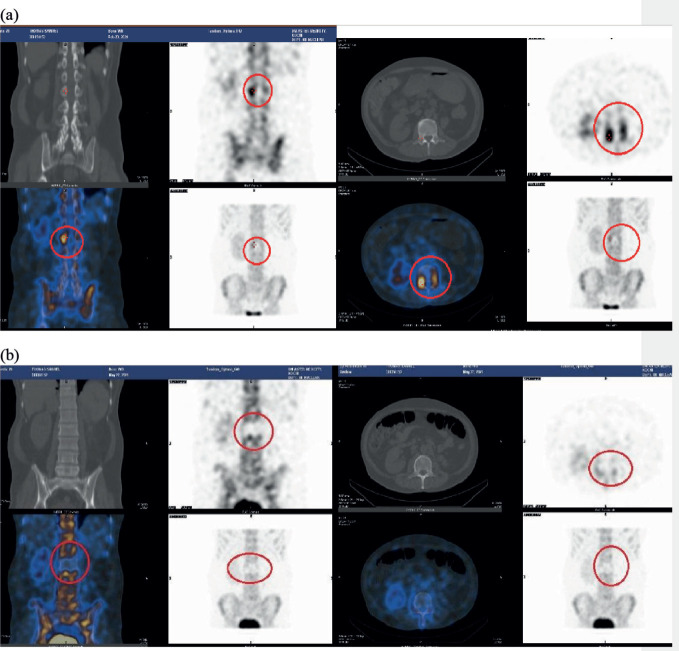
(a): SPECT images in metastatic RCC showing increased tracer uptake over the L2 pedicle on the right. (b): SPECT images 3 months after intracranial FSR, showing no tracer uptake over the L2 pedicle on the right.
